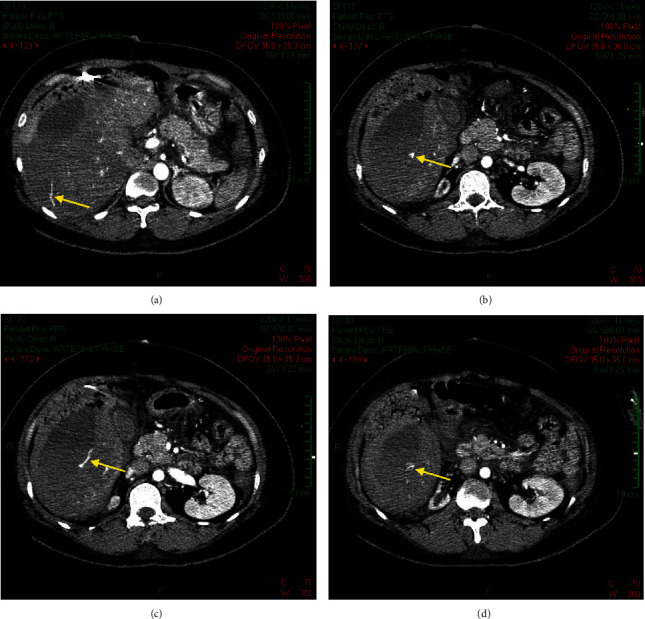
Subhepatic hematoma and subscapular active bleeding on CT scan at ED. (a, c) Active bleeding signs (yellow arrow) and (b, d) other extravascular contrast (yellow arrow). CT: computed tomography; ED: emergency department.
